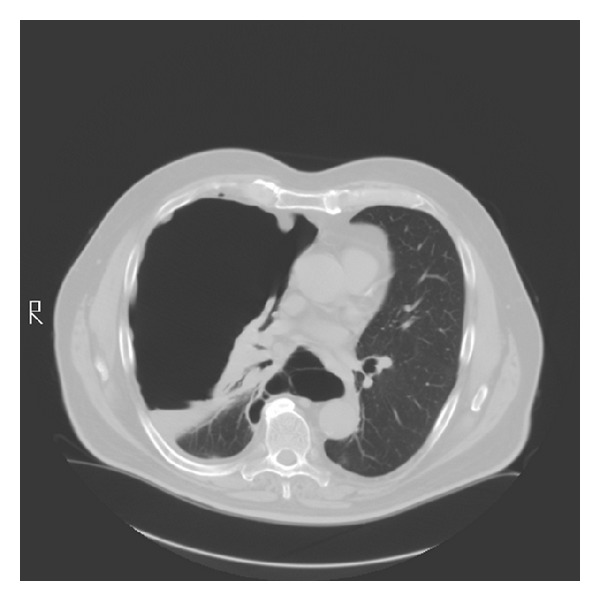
Chest CT scan in August 2008 showing a large right pneumothorax with atelectasis of the right upper and middle lobes.
